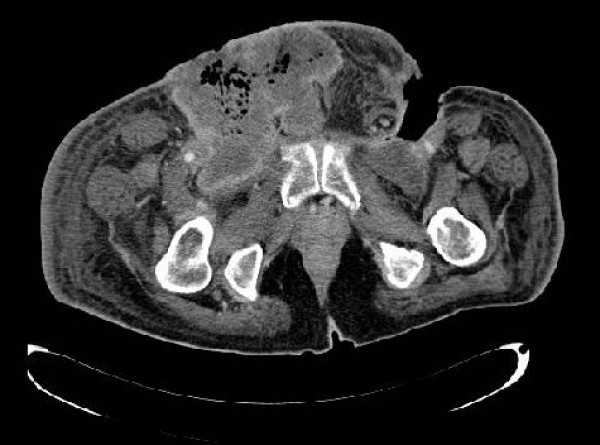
Several large necrotic adenopathies in inguinal, scrotal and pubic area (CT scan).
